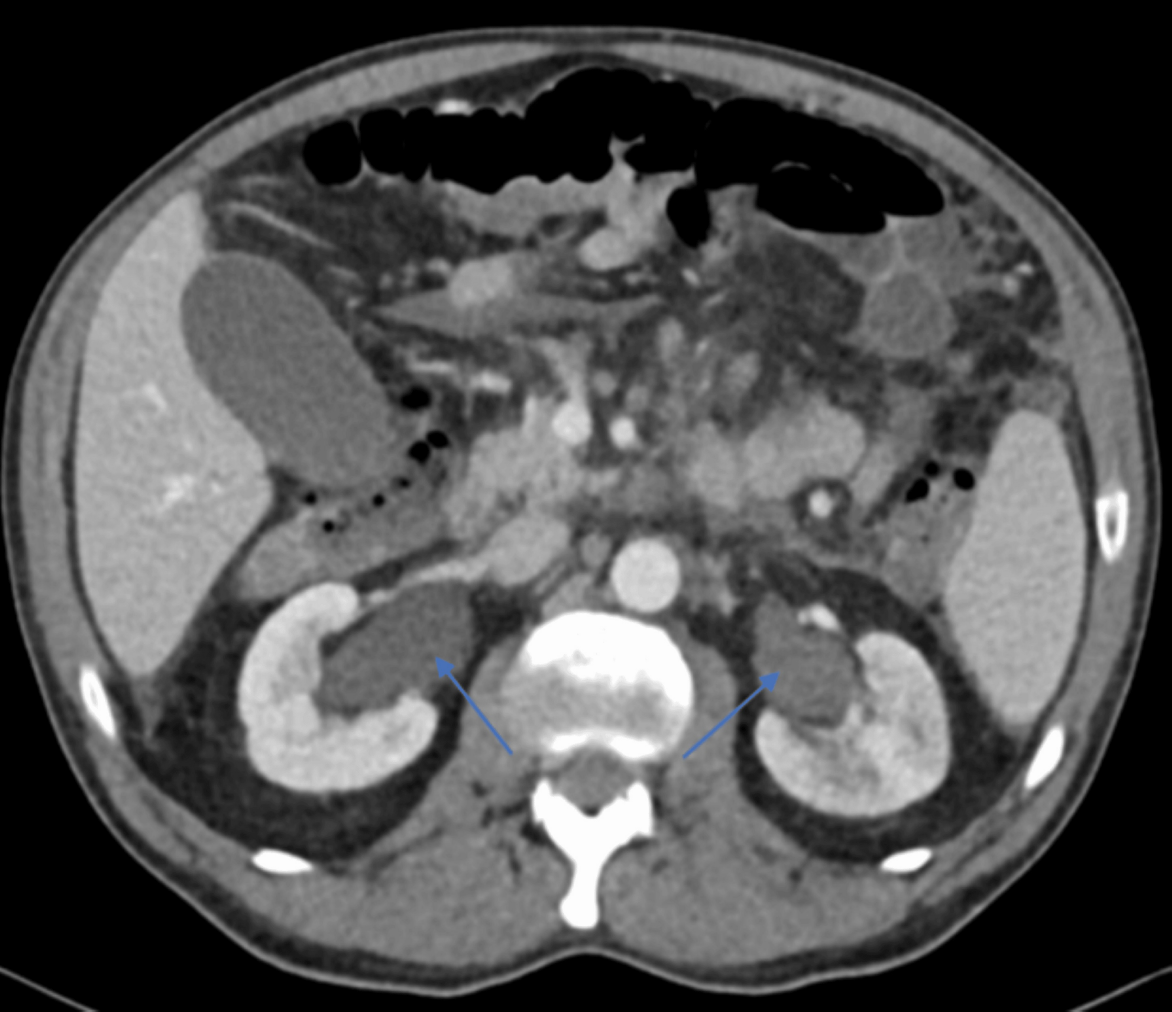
A CT abdomen and pelvis with contrast CT reveals moderate bilateral hydronephrosis (depicted by blue arrows).
